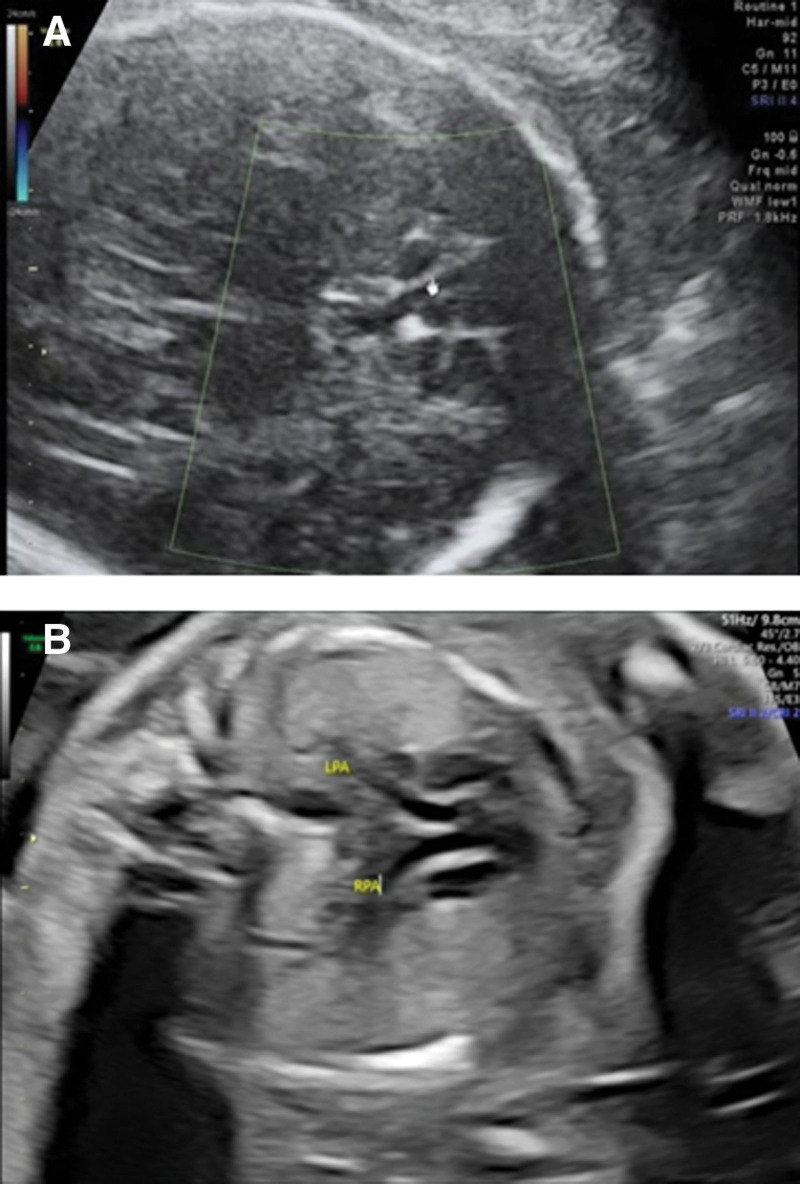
Typical ultrasound performance of the cases. (A) The ultrasound image of Case 1 showed the subependymal cyst. (B) The ultrasound image of Case 2 showed the persistent left superior vena cava.
